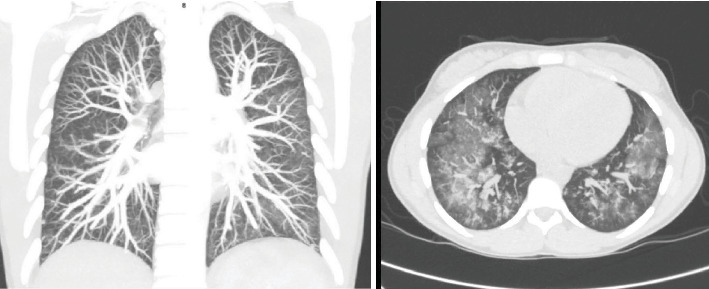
Computed tomography of the chest showing bilateral ground glass opacities.
